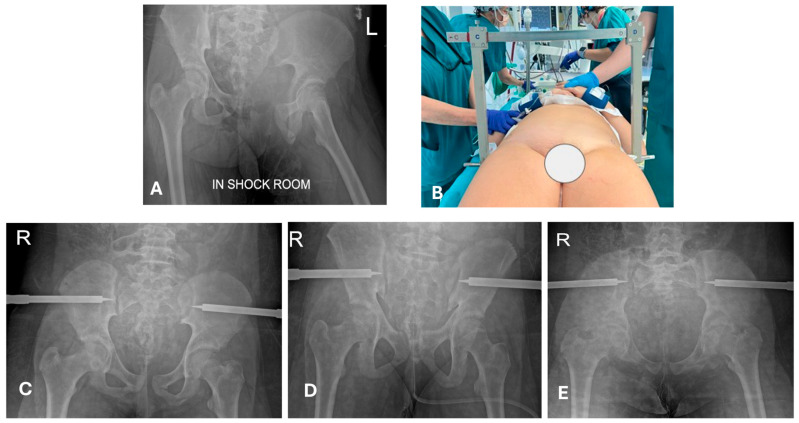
A 12-year-old girl, after high-energy trauma (motocross accident: direct frontal collision with a tree during a jump). (A) Unstable open pelvic ring fracture. The patient was hemodynamically unstable upon admission. The exposure of the fracture was in a cavity (the left pelvic ramus protruded through the vagina), which is a lesion that might be easily missed during the primary evaluation without a systematic approach and exposure of the patient. (B) Pelvic fixation in an emergency with a pelvic C-clamp. (C–E) Postoperative radiographs (antero-posterior and outlet and inlet views, respectively). The patient survived and followed definitive treatment in the following days. Courtesy of Dr Garcia Parra C. and Pelis A (Papa Giovanni XXIII Hospital, Bergamo), with permission.
